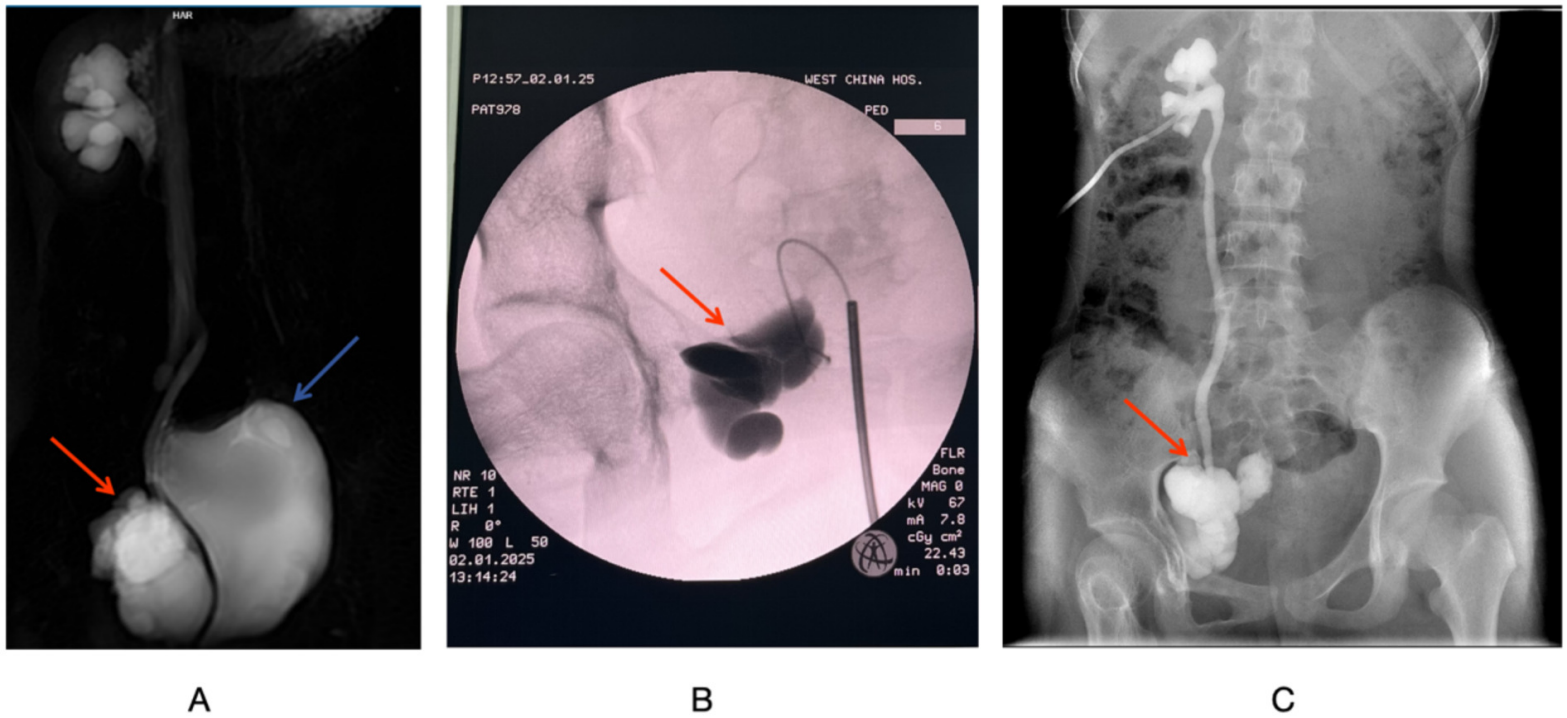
(A) MRI T2-weighted coronal imaging of the urinary system. (B) Intraoperative retrograde pyelography via the ureter. (C) Right side antegrade pyelography. The red arrow indicates the DT, and the blue arrow points to the bladder.
